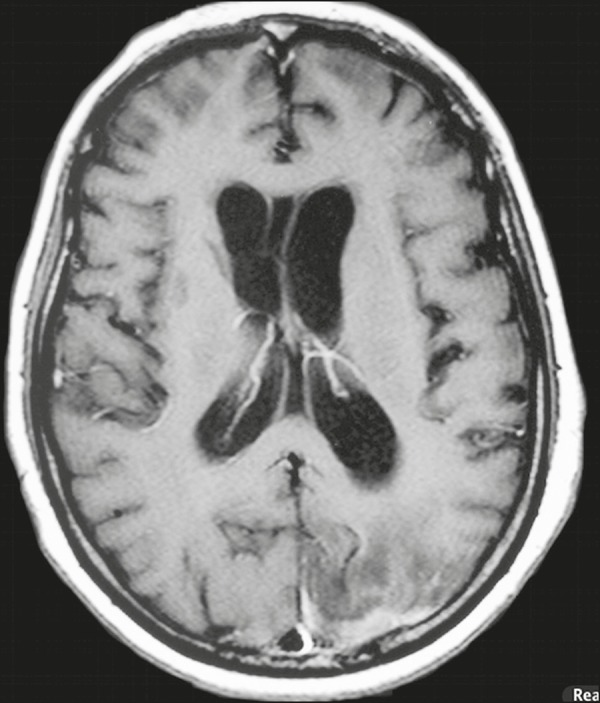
Case 9.* Contrast-enhanced axial T1-weighted image showing mild
